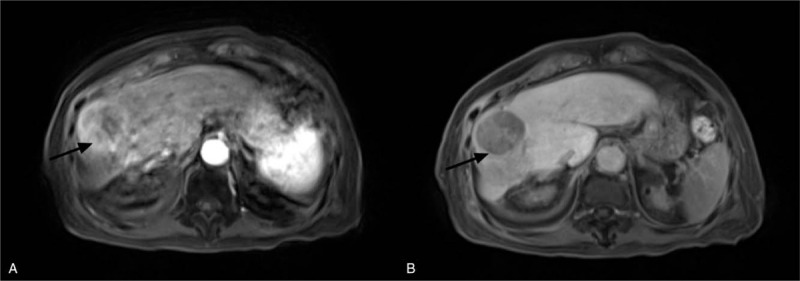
Fifteen months after the CyberKnife procedure (May 2019), contrast-enhanced MRI well-defined showed the local failure of S5/8. The lesion enlarged to 4.9 cm × 4.7 cm and showed arterial phase enhancement (A), followed by a washout in the venous phase (B).
